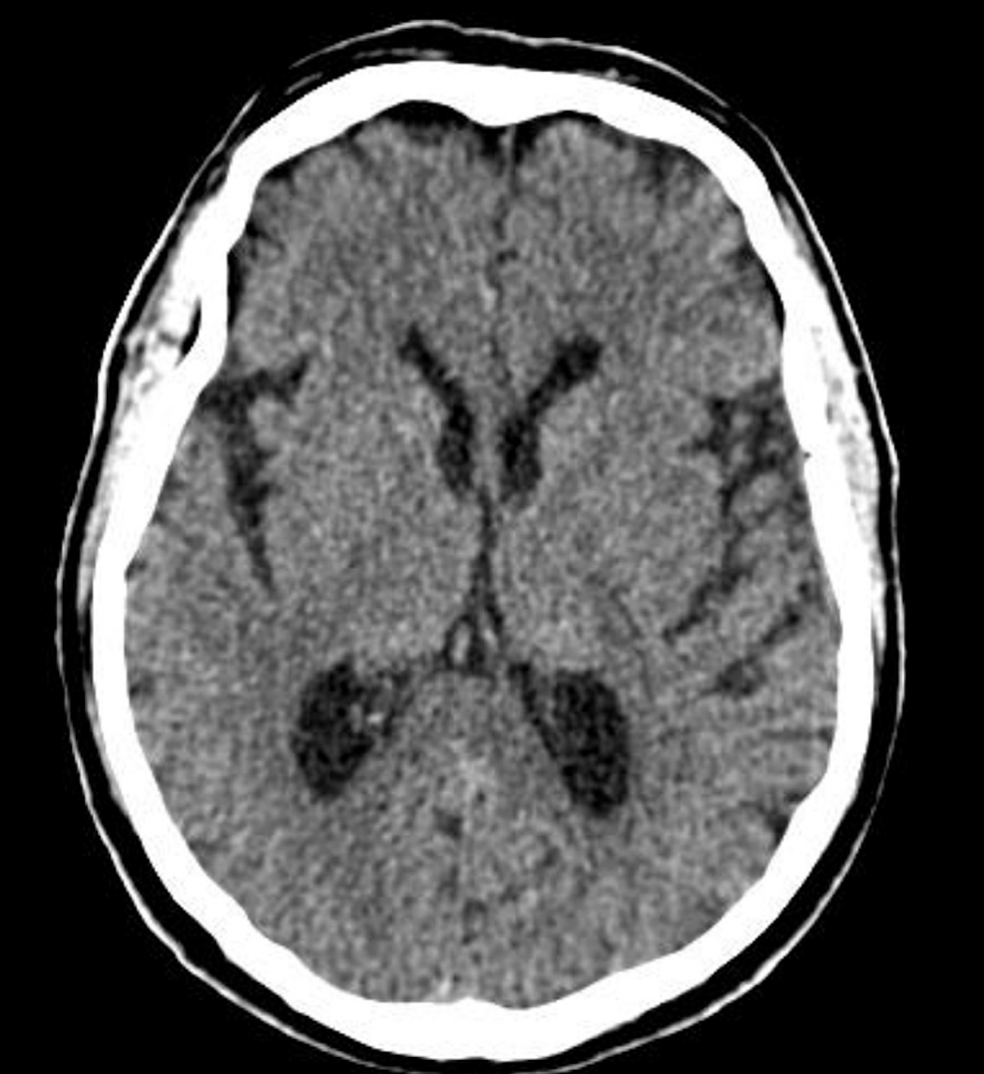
Computerized tomography (CT) of the head showing moderate parenchymal atrophy and microangiopathy. There was no sign of any acute pathology.
